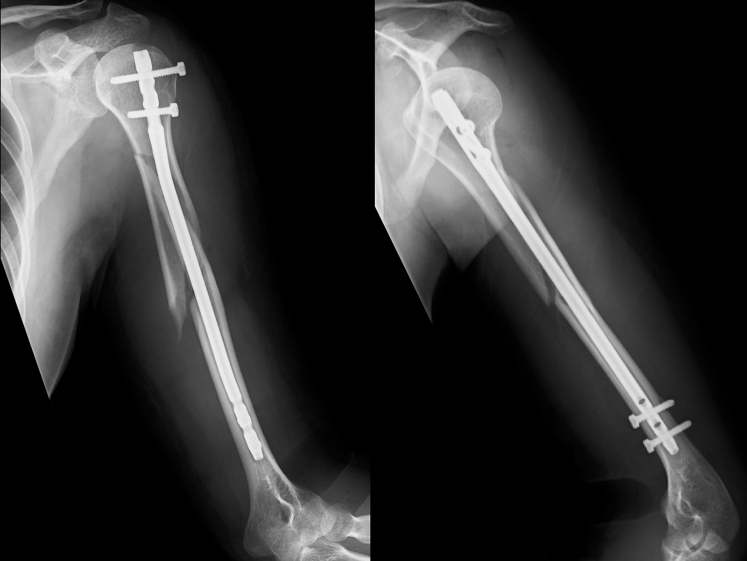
Plain radiographs after osteosynthesis.
